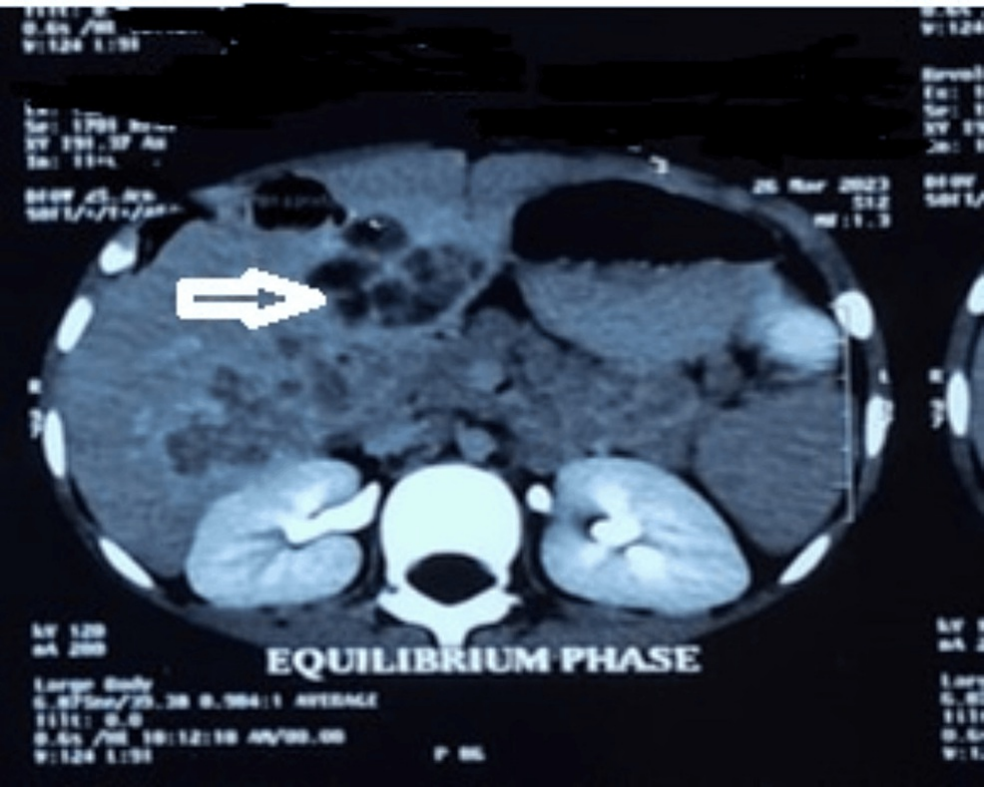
A CT scan confirmed the presence of multiple cystic hepatic lesions with multiloculation and some air density. Marginal enhancement with septations, septal enhancement, and mildly reactive adjacent parenchymal tissue
